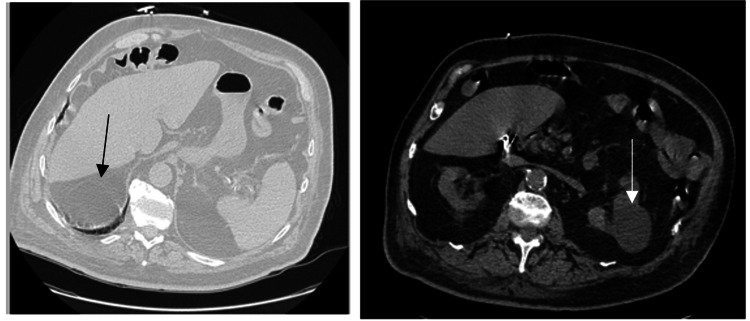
CT imaging of the abdomen and pelvis; the arrow highlights a liver cyst on the left and a renal cyst on the right
